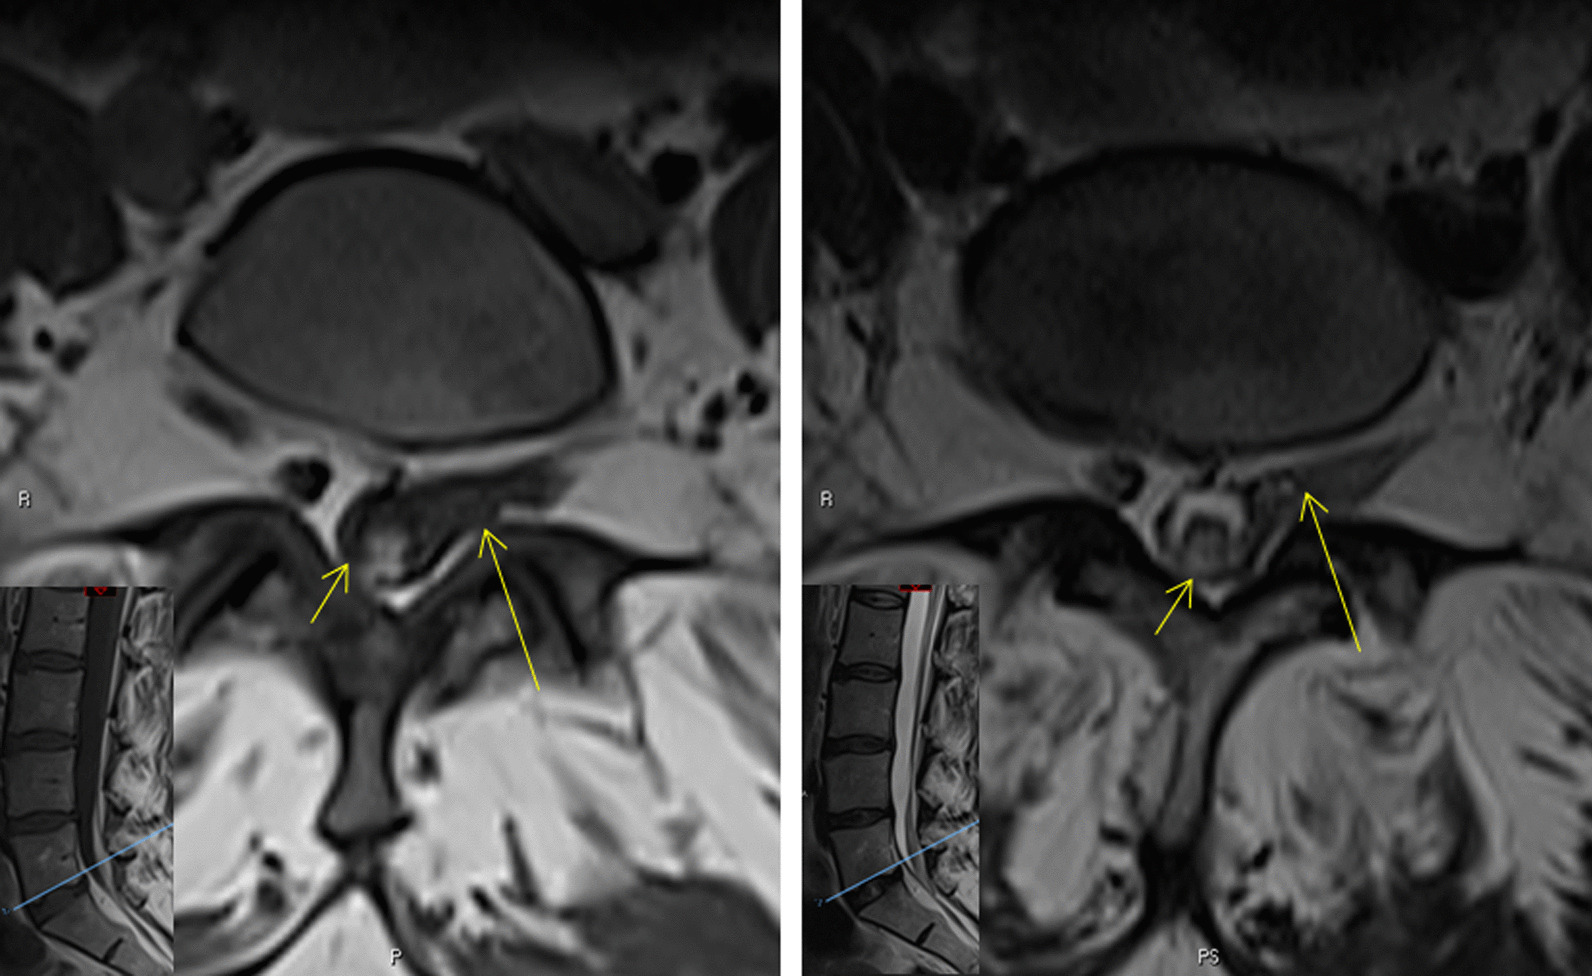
Initial images: Axial magnetic resonance imaging lumbar spine T1-weighted (left) and T2-weighted (right). On the axial images, in addition to the intrathecal blood clot (short arrow), there is perineural inflammatory stranding (long arrow) around the exiting left S1 nerve root fascicles
